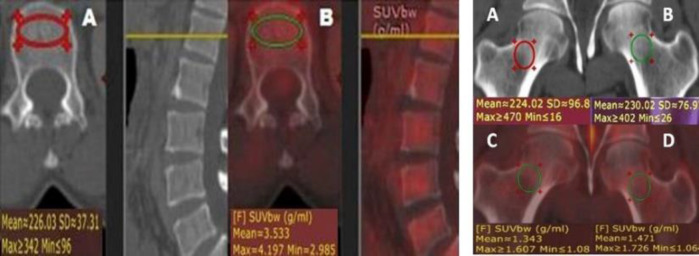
Examples of methods of calculation of vertebral and femoral neck CT HU attenuation and SUV_max_ measurements
